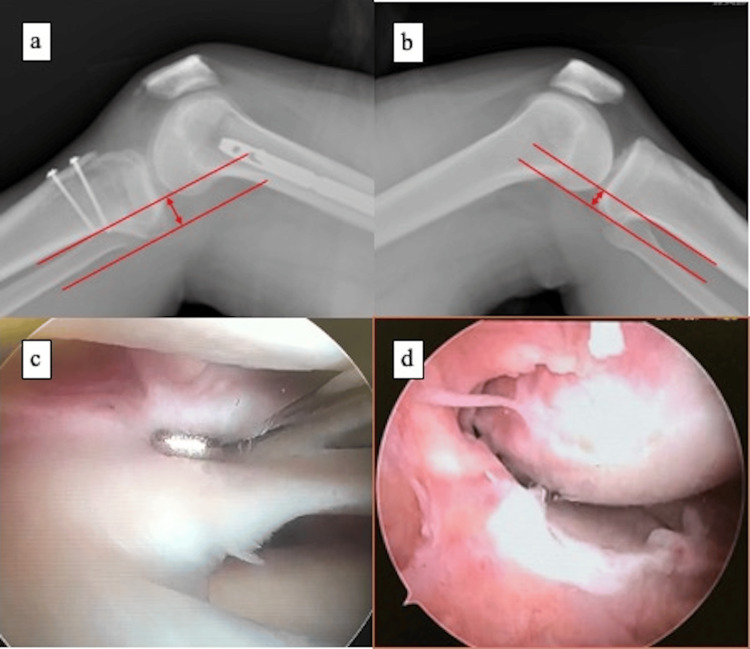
Pre-second-stage radiographs and intraoperative findings (a-b) Gravity-sag views demonstrating a 6-mm side-to-side difference between the affected and unaffected knees. Dashed lines indicate the posterior cortical reference lines used to measure posterior tibial translation, and the double-headed arrow indicates the measured side-to-side difference. (c) Arthroscopic confirmation of healing of the repaired lateral meniscus. (d) Arthroscopic visualization of posterior cruciate ligament injury.
